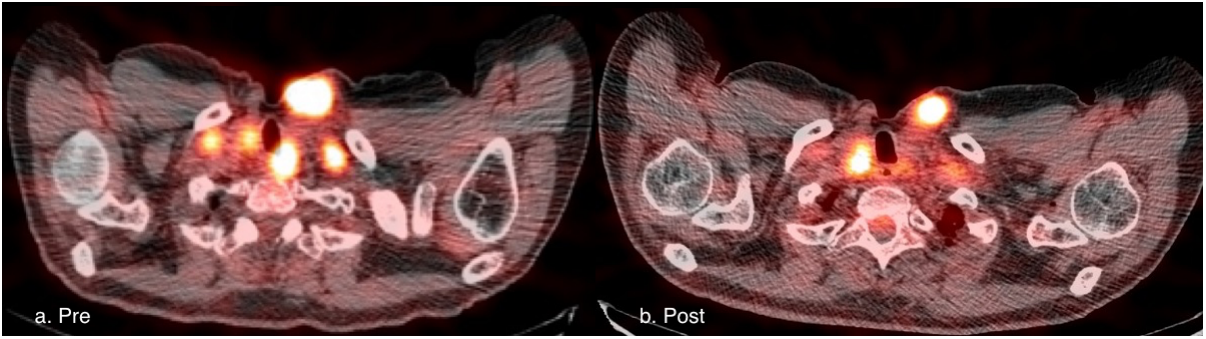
Fusion PET/CT image at baseline (A.Pre) and after 4 weeks of therapy with pazopanib (B.Post) in a patient with well-differentiated thyroid cancer. Post-pazopanib scan demonstrate some improvement in FDG-avid disease, although overall this patient had stable disease (Patient 6). FDG, ^18^F-fluorodeoxyglucose; PET, positron-emission tomography.
